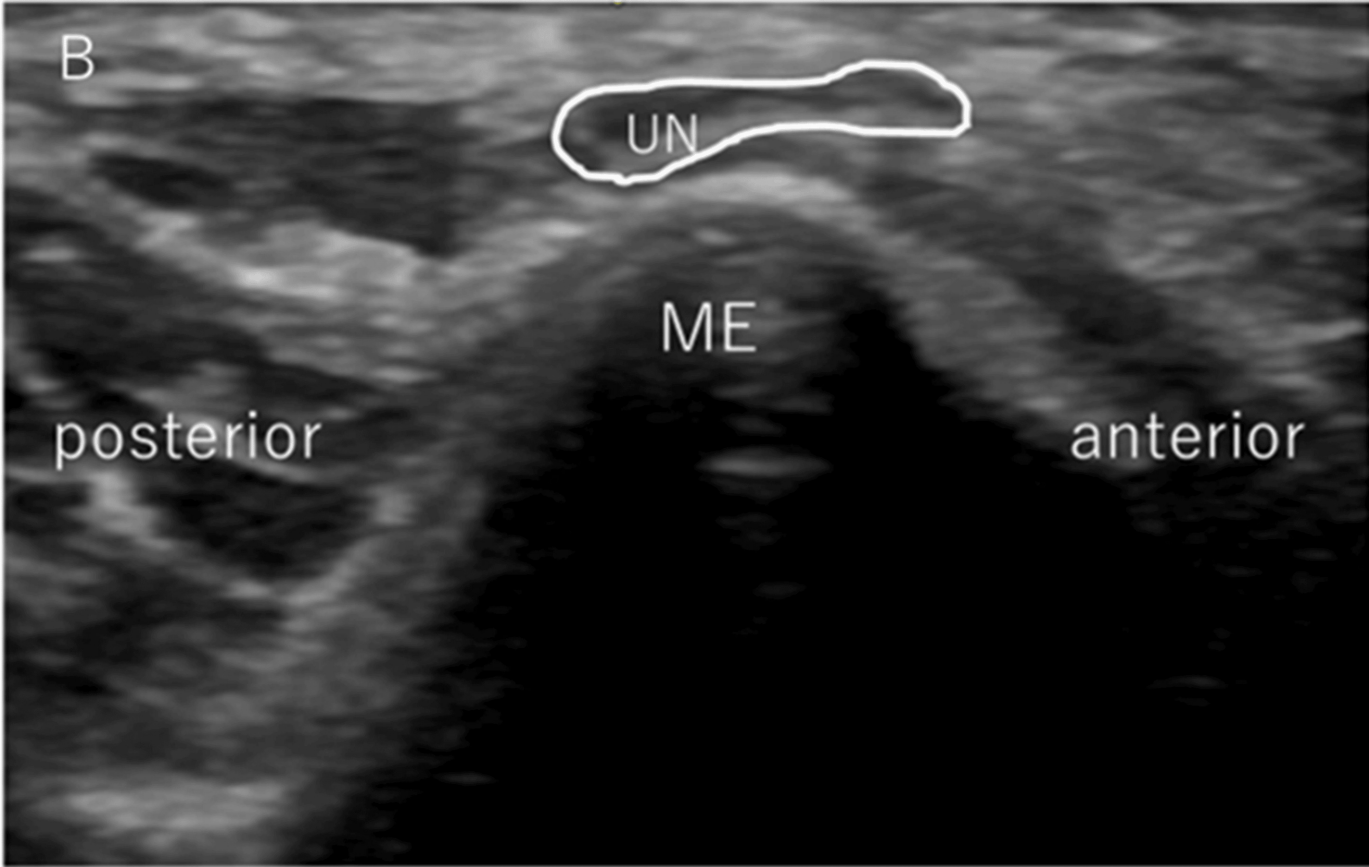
Assessment of ulnar nerve instability: subluxation type Transverse US images of the ulnar nerve show subluxation. ME: medial epicondyle; UN: ulnar nerve; US: ultrasonography
